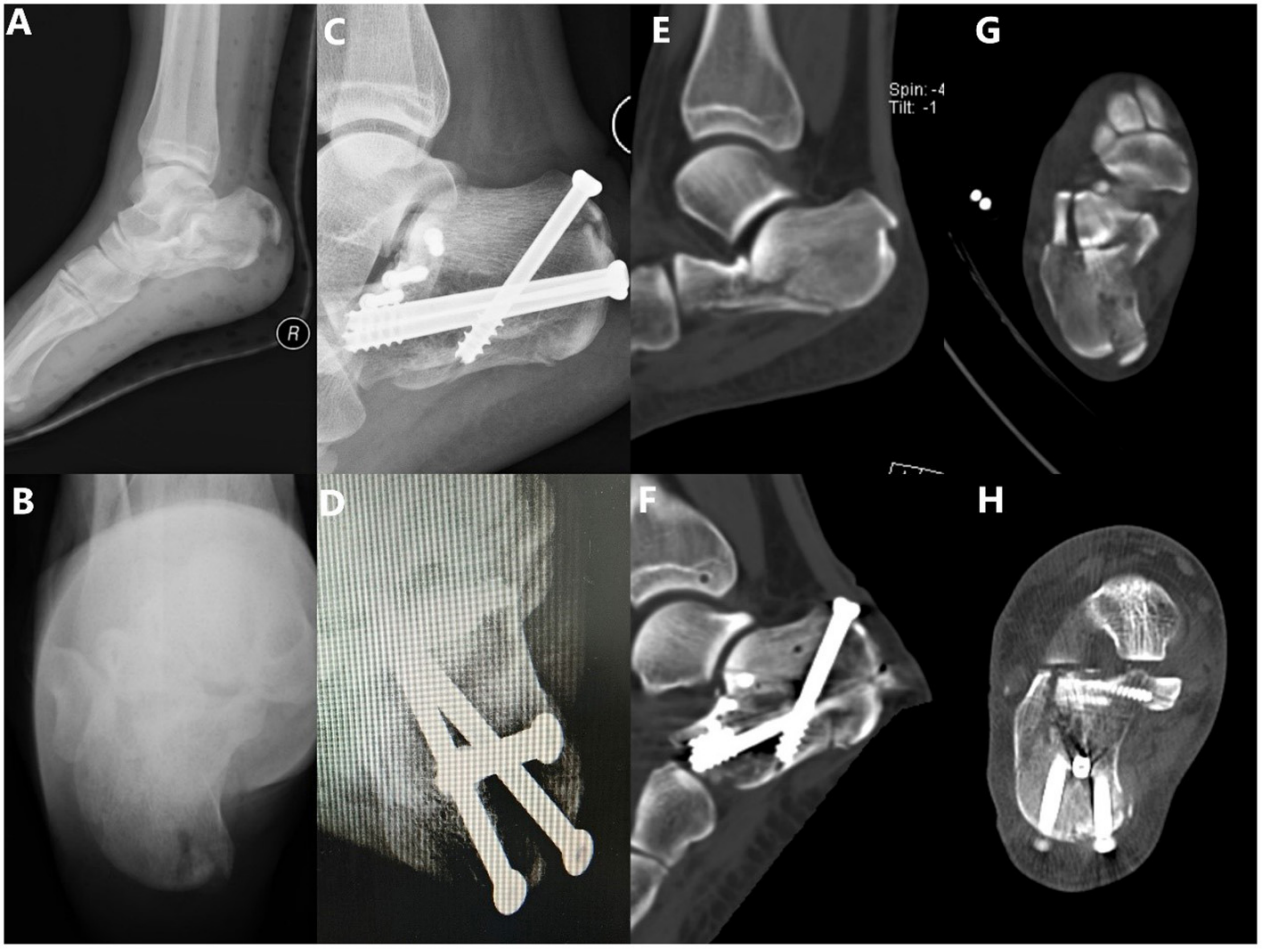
A 19-year-old female patient who fell from a height, resulting in a tongue-type intra-articular fracture of the calcaneum. (A) Preoperative lateral X-ray and (B) preoperative axial X-ray presenting an intra-articular tongue-type calcaneal fracture with subtalar articular surface collapse, loss of Böhler's angle and Gissane's angle, significantly decreased calcaneal height/length, and significantly increased calcaneal width. (C) Postoperative lateral X-ray showing the reduction of calcaneal length, height, Böhler's angle, and Gissane's angle. (D) Postoperative axial X-ray showing the dissatisfactory restoration of the calcaneal varus. (E,G) The preoperative computed tomography (CT) scan shows a Sanders type II fracture. (F,H) Postoperative CT scan shows the reduction of the articular surface was satisfactory, but the restoration of the calcaneal width was dissatisfactory.
